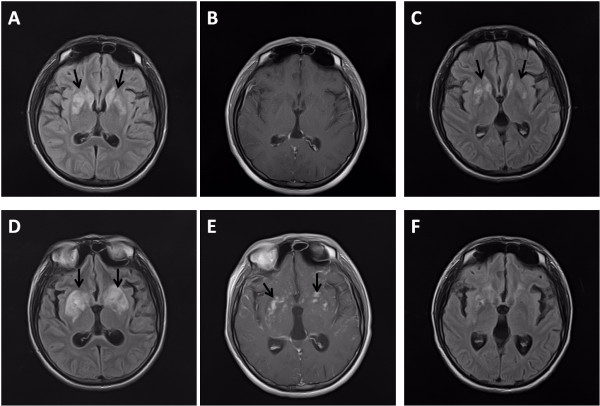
Brain MRI of the patient. Axial T2-FLAIR imaging obtained soon after admission showed abnormal hyperintensities in the bilateral basal ganglia (arrows in A) without enhancement (B). After 7 weeks on anti-cryptococcal therapy, axial T2-FLAIR images showed partial resolution of the lesion (arrows in C). Twenty-five weeks after initiation of anti-retroviral therapy and while on fluconazole maintenance therapy, axial T2-FLAIR images showed abnormal hyperintensities in bilateral basal ganglia again (arrows in D) with irregular patchy enhancement (arrows in E). After 19 days on corticosteroids and intensified anti-cryptococcal therapy, T2-FLAIR images showed near complete resolution of brain lesions (F).
